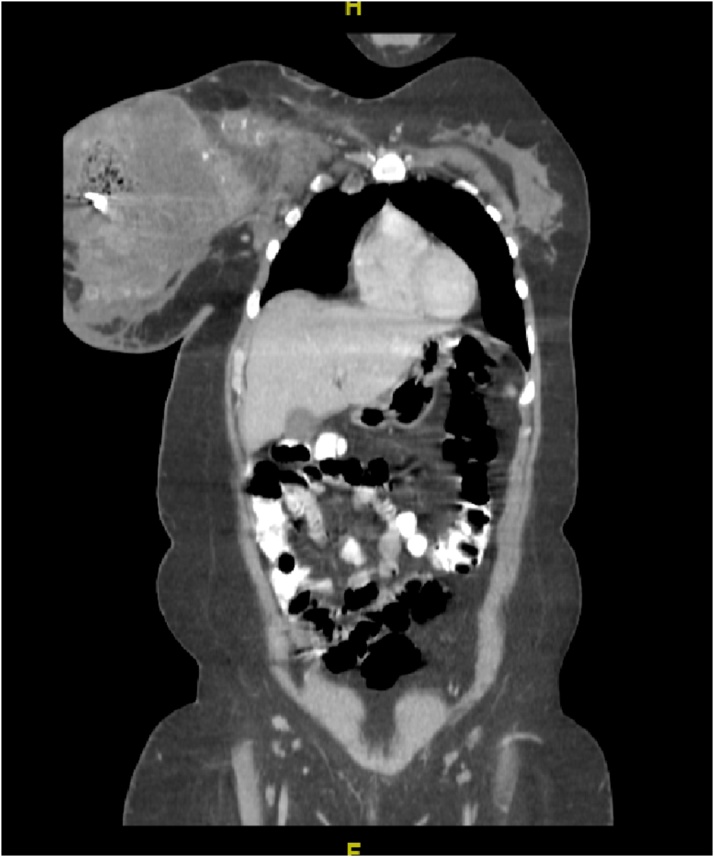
CT-Scan chest coronal section.
